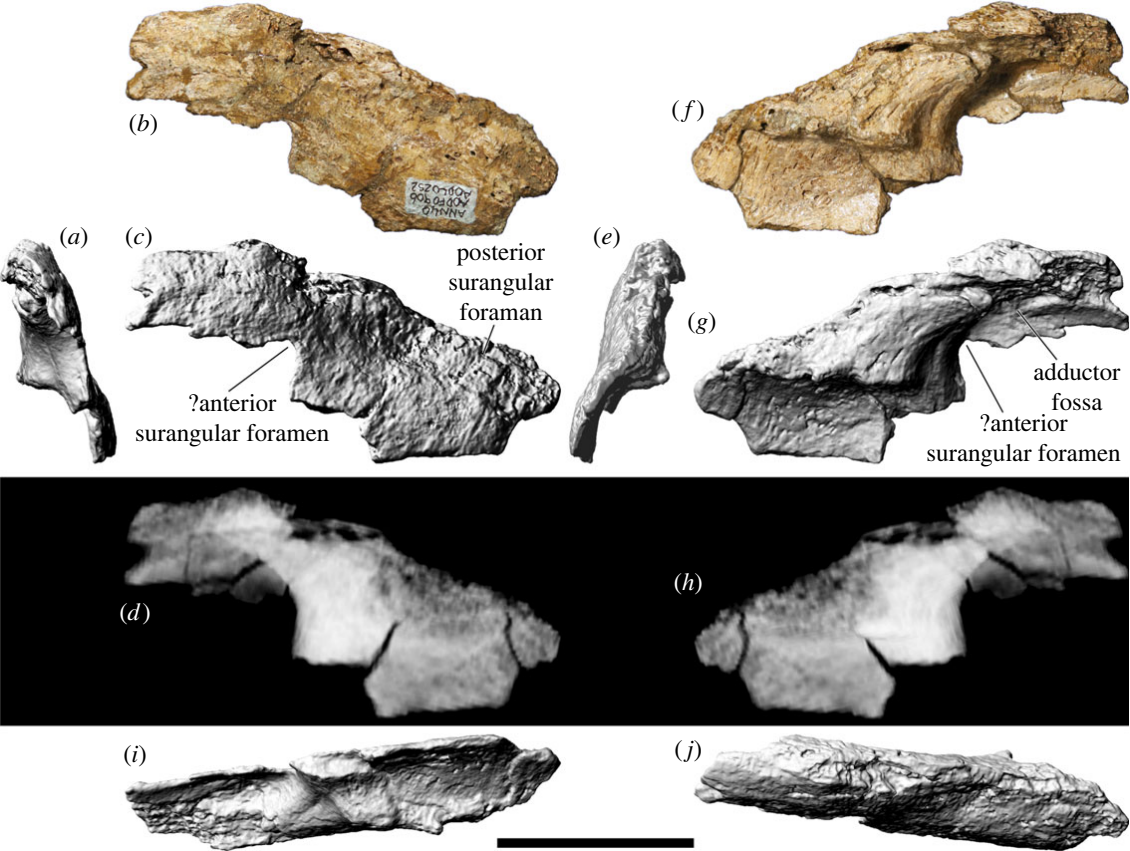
Diamantinasaurus matildae* referred left surangular (AODF 0906). (*a–j*) Left surangular in (*a*) anterior, (*b–d*) lateral, (*e*) posterior, (*f–h*) medial, (*i*) ventral and (*j*) dorsal views. (*a*), (*c*), (*e*), (*g*) and (*i–j*) are three-dimensional models derived from surface scans; (*b*) and (*f*) are photographs; and (*d*) and (*h*) are three-dimensional models derived from CT scans. Scale bar = 50 mm.
